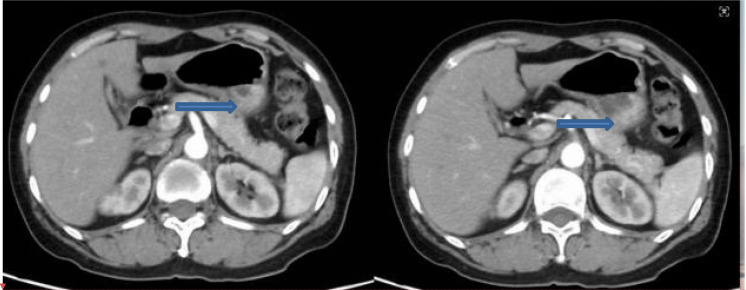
Representative axial contrast-enhanced abdominal CT images obtained at admission for recurrent disease, showing thickening of the gastric wall along the greater curvature with heterogeneous enhancement.
